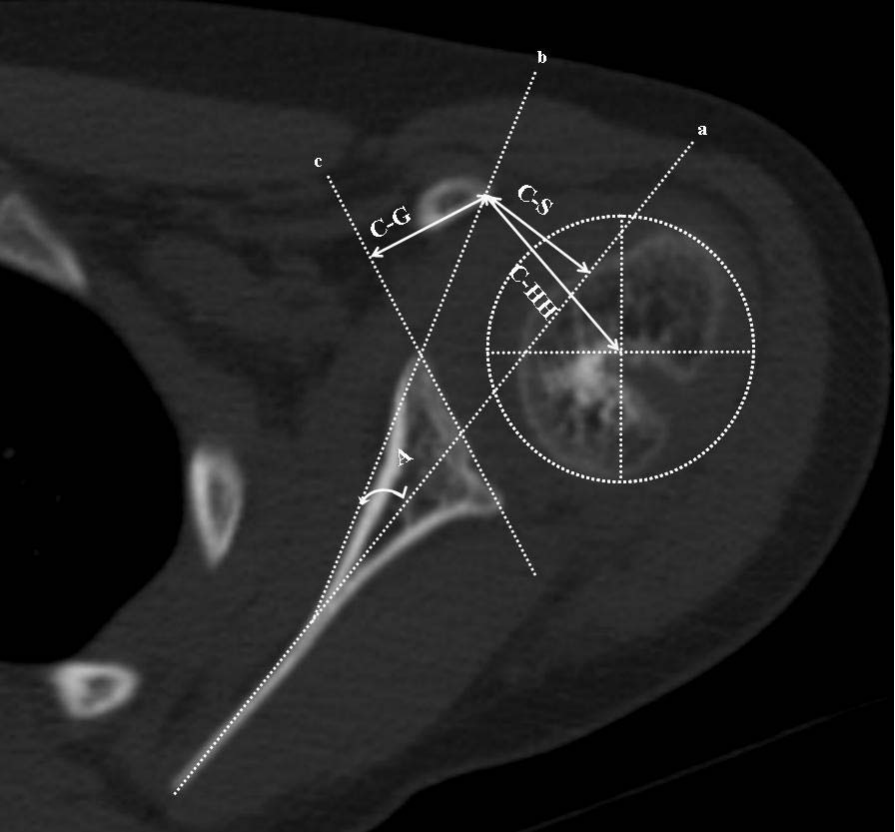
Representative axial CT image of the affected shoulder from a patient with obstetric brachial plexus injury. Line "a" is the scapular line. Line "b" starts at the most anterior portion of the glenoid labrum and runs tangentially to the most prominent aspect of the coracoid tip. Line "c" represents the glenoid plane. Angle "A" formed between lines "a" and "b" is the coracoscapular angle. C-G represents the distance of coracoid overlap. C-HH represents the coracohumeral distance. C-S represents the coracoscapular distance.
